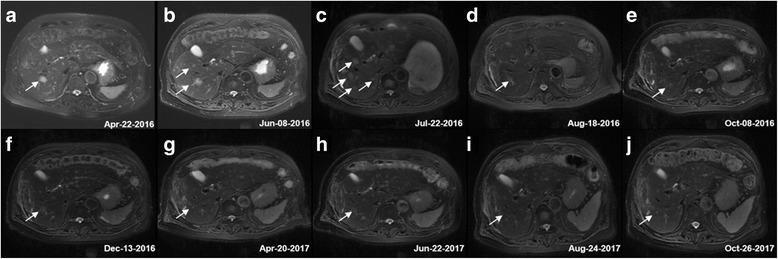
Abdomen MRI T2WI changes in liver lesions. a Apr-22-2016 MRI showing multiple hepatic lesions found. b Jun-08-2016 MRI showing hepatic lesions increase in number and size. c Jul-22-2016 MRI showing new lesions emerge after RFA. d Aug-18-2016 MRI showing tumor up to PR after first cycle of pazopanib plus PD-1 inhibitor and RAK cell. e-j From Oct-08-2016 to Oct-26-2017 MRI showing tumor stable disease. Arrows indicate the lesion. MRI, magnetic resonance imaging; PR, partial response; RAK cell. RetroNectin-activated killer cells
